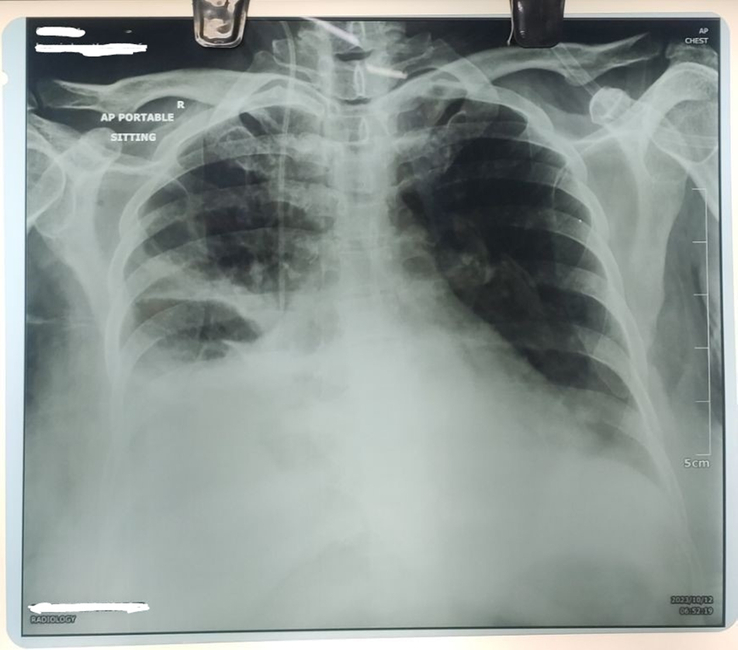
Chest radiograph anteroposterior view showing bilateral pleural effusion prior to pleural tapping.
